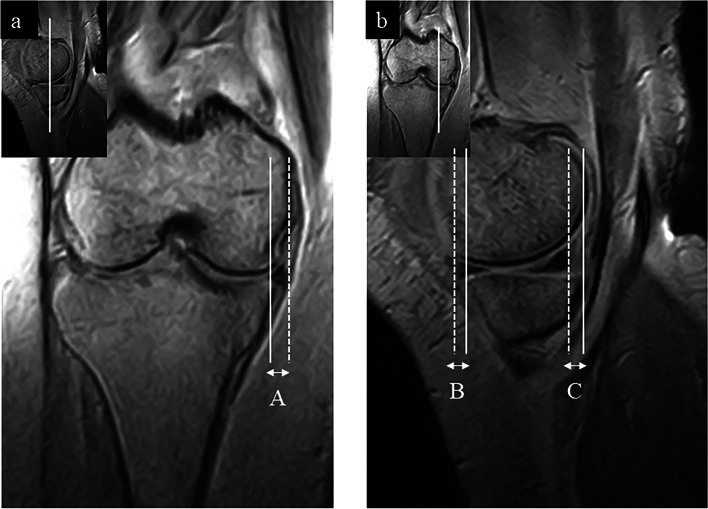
Evaluation of the meniscal extrusion on MRI. a Coronal plane evaluation image and (b) sagittal plane evaluation image. A Medial extrusion of the medial meniscus, (B) anterior extrusion, and (C) posterior extrusion of the medial meniscus. MRI, magnetic resonance imaging
